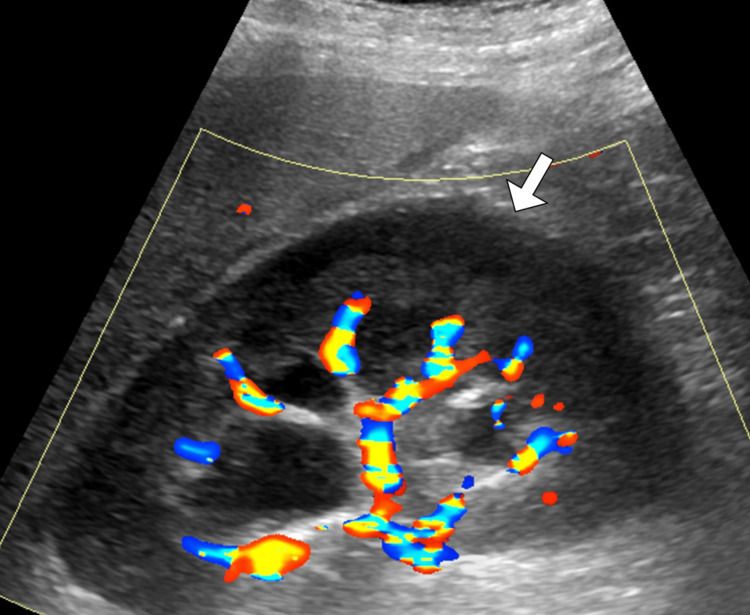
Color Doppler ultrasound of left perirenal collection. Color Doppler ultrasound image of the left kidney shows the previously identified perirenal collection (arrow) without internal vascularity. The absence of Doppler flow helps exclude a vascular lesion, supporting the diagnosis of a perirenal hematoma.
